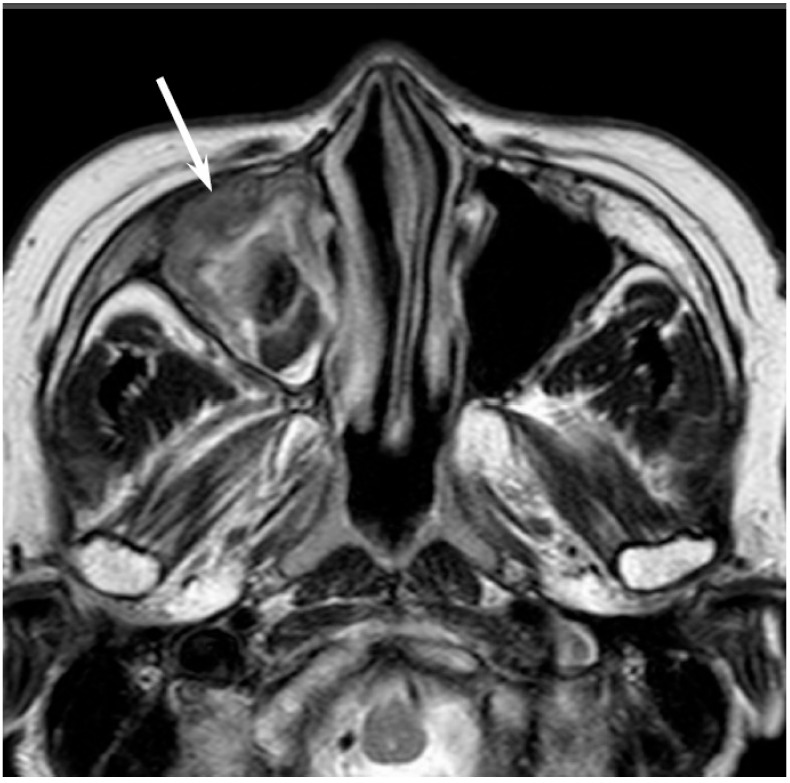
Malignant peripheral nerve sheath tumor of the right maxillary sinus. T2-weighted image showing a heterogeneously hypo- to hyperintense lesion (arrow).
